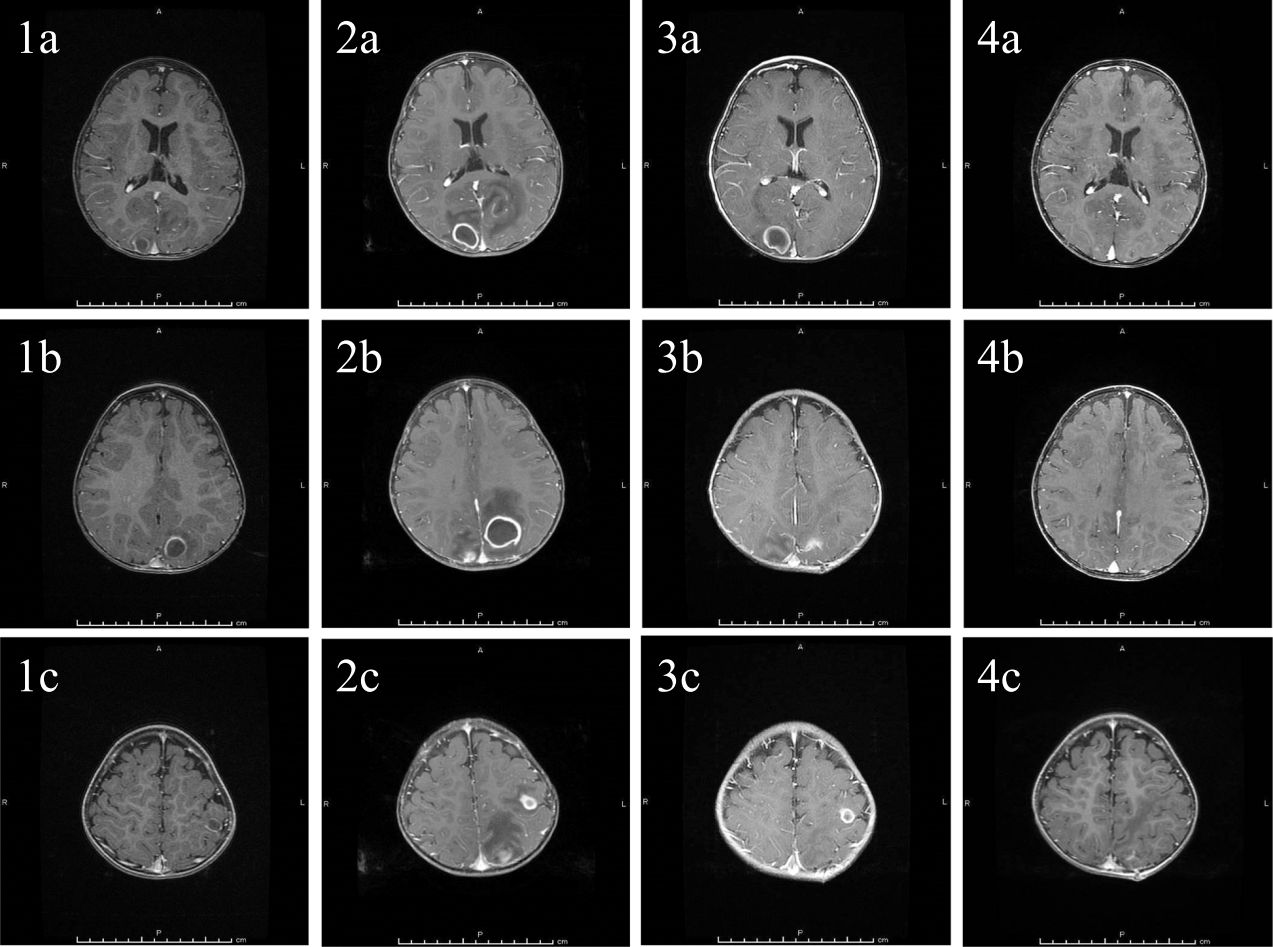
Clinical course of the lesions as seen on gadolinium-enhanced T1-weighted magnetic resonance images. Brain abscesses were located in the right occipital lobe (1a–4a), left occipital lobe (1b–4b), and left parietal lobe (1c–4c) on the day of admission (1a–1c), at 8 days after admission (2a–2c), at 13 days after surgical aspiration (3a–3c), and at 4.5 months after onset (4a–4c). Each brain abscess was enlarged after admission (2a–2c), reduced after surgical aspiration (3a–3c), and eventually disappeared (4a–4c)
